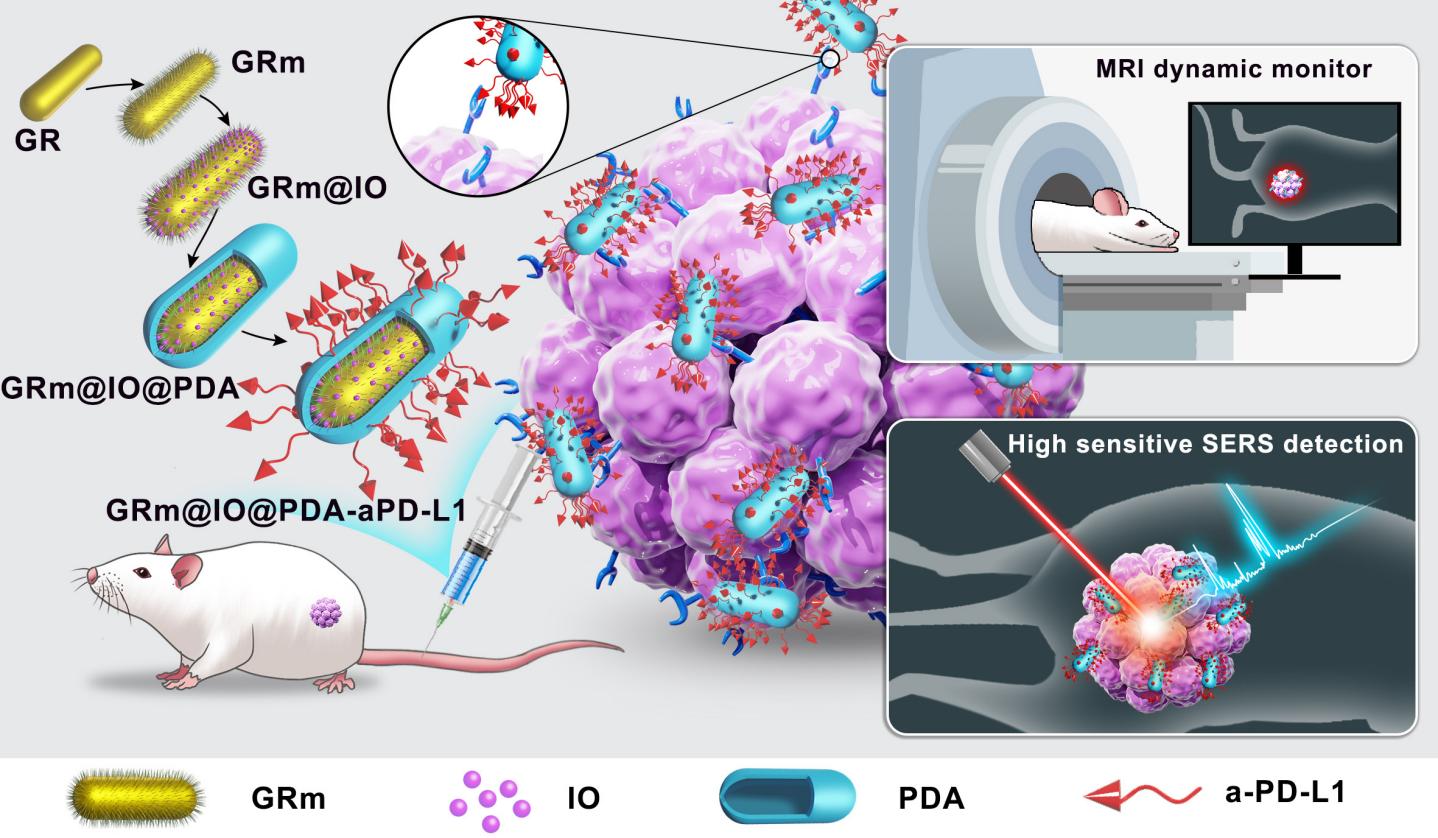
Design of the programmed cell death ligand-1 (PD-L1) targeted SERS-MRI dual-modal bioprobes (GRm@IO@PDA-aPD-L1) for specific and sensitive detection of PD-L1 expression in tumor.
